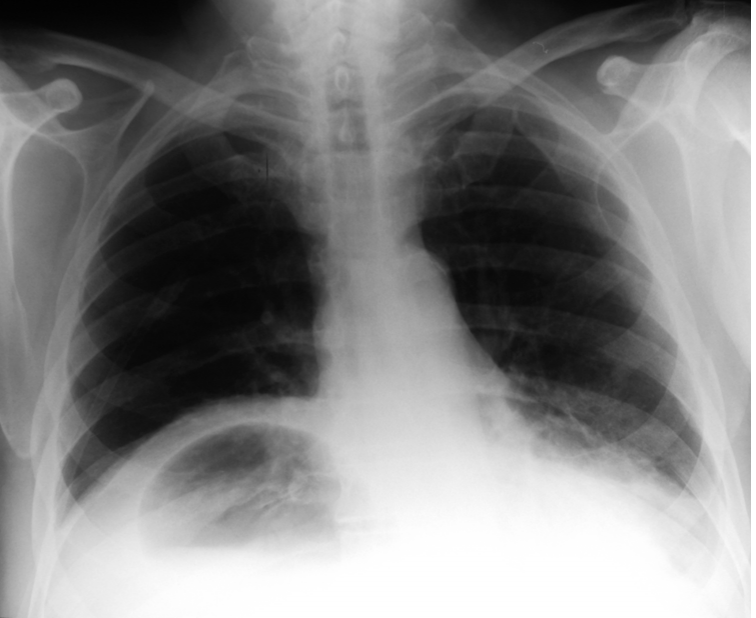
Chest x-ray on admission showing an unusual air fluid level in the lower chest.
